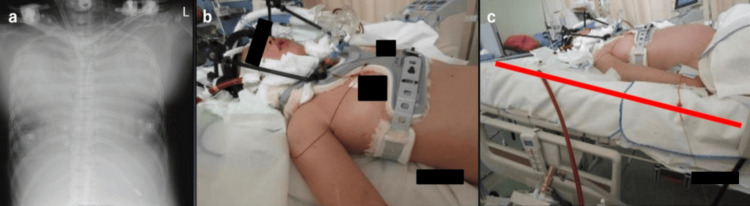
V-V ECMO management under the halo vest (a) Chest X-ray after ECMO initiation showing bilateral infiltrates. A cannula is placed in the right internal jugular vein. (b) Clinical photo showing generalized edema and limited thoracic expansion due to the halo vest. (c) Bed tilted to a 15-30° reverse Trendelenburg position, improving tidal volume (red line). ECMO, extracorporeal membrane oxygenation; V-V ECMO, veno-venous extracorporeal membrane oxygenation
